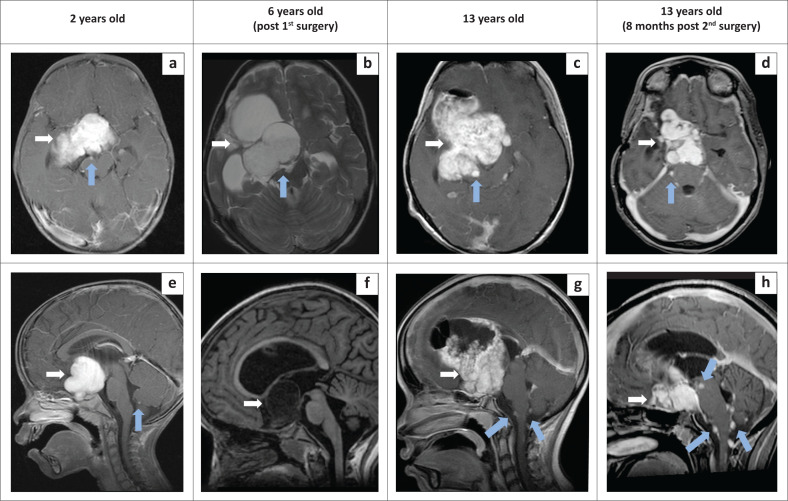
Series of axial (a, b, c, d upper row) and sagittal (e, f, g, h lower row) MR imaging over 11 years at age 2, age 6 and age 13. White arrow: suprasellar tumour. Blue arrow: leptomeningeal nodule. (a, e) Contrasted MRI brain at age 2 shows a large, solid, enhancing suprasellar mass with small enhancing leptomeningeal nodules at the right interpeduncular cistern (a) and anterior to the cerebellar vermis (e). (b, f) Repeat MRI brain at age 6 after partial tumour resection shows a smaller suprasellar mass causing obstruction of the right lateral ventricle. The leptomeningeal nodule at the right interpeduncular cistern is still present and appears isointense on T2WI (b). Contrast administration was declined at this time. (c, g) Repeat MRI brain at age 13 revealed that the residual suprasellar mass had increased in size and the leptomeningeal nodules increased in size and number in the right interpeduncular cistern (c) and anterior to the cerebellar vermis with new nodules at the craniocervical junction (d). (f, h) Repeat MRI at 8 months post surgery showed a decrease in the size of the suprasellar mass with an increase in the size and number of leptomeningeal nodules.
